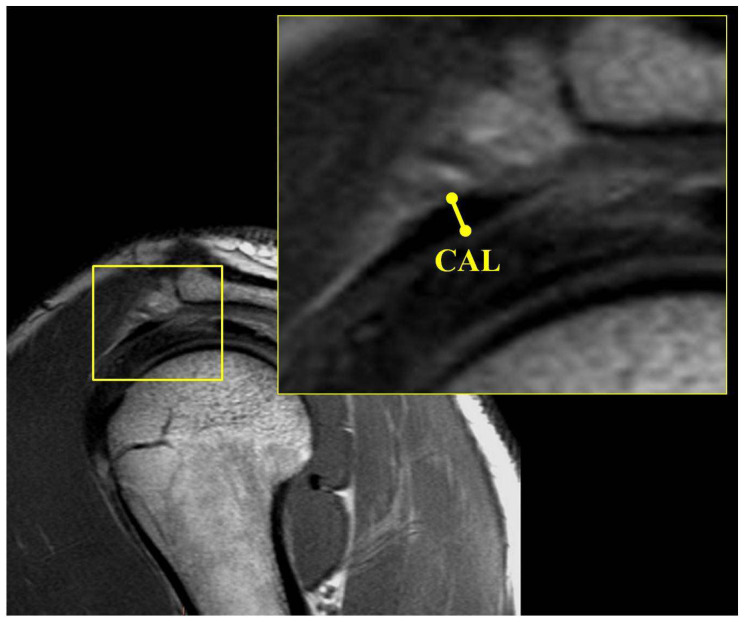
Sagittal MRI of shoulder showing measurement of coracoacromial ligament (CAL).
